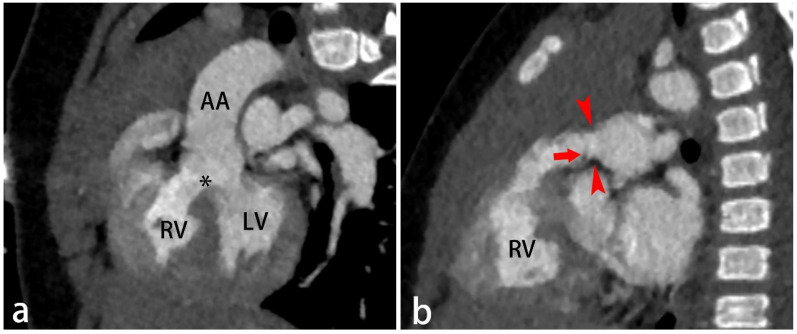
A 5-month-old boy with tetralogy of Fallot. CT angiography oblique image shows a large subaortic ventricular septal defect ((a), black asterisk), with overriding of the ascending aorta above the ventricular septal defect. The pulmonary valve shows a dome-shaped appearance ((b), red arrow), and supravalvular pulmonary stenosis is seen ((b), red arrowheads). The right ventricular wall is hypertrophied. AA, ascending aorta; RV, right ventricle; LV, left ventricle.
